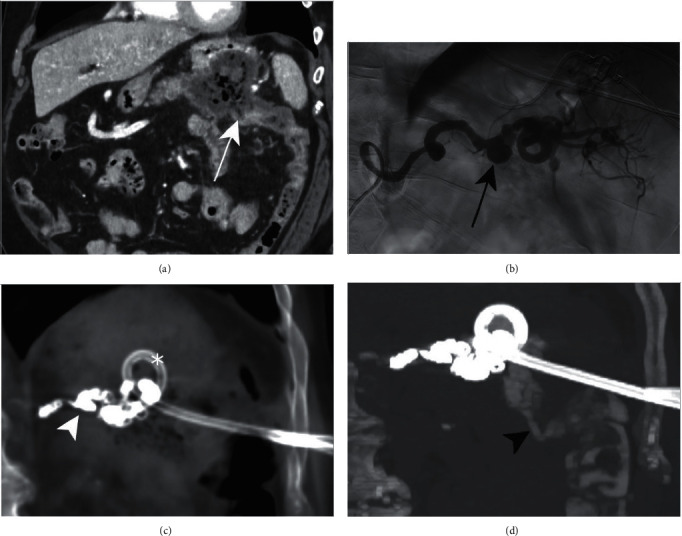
(a) Coronal reformatted contrast-enhanced CT demonstrates a large necrotic tumor in the pancreatic tail with suspicion of a fistula extending to the left colic angle (white arrow). (b) Digital subtraction angiography of the splenic artery shows caliber irregularities of the proximal and middle thirds of the vessel and a 15 mm diameter pseudoaneurysm (black arrow) of the middle third of the artery, without contrast extravasation; in the normal segments, the splenic artery measures 4–5 mm of diameter. Coronal reformatted CT with maximal intensity projection before (c) and after (d) positive contrast injection through the drainage catheter (asterisk) placed in the necrotic pancreatic mass reveals a pancreatico-colic fistula (black arrowhead) responsible for a concomitant opacification of the left colon. Images were acquired after endovascular embolization with coils (white arrowhead).
